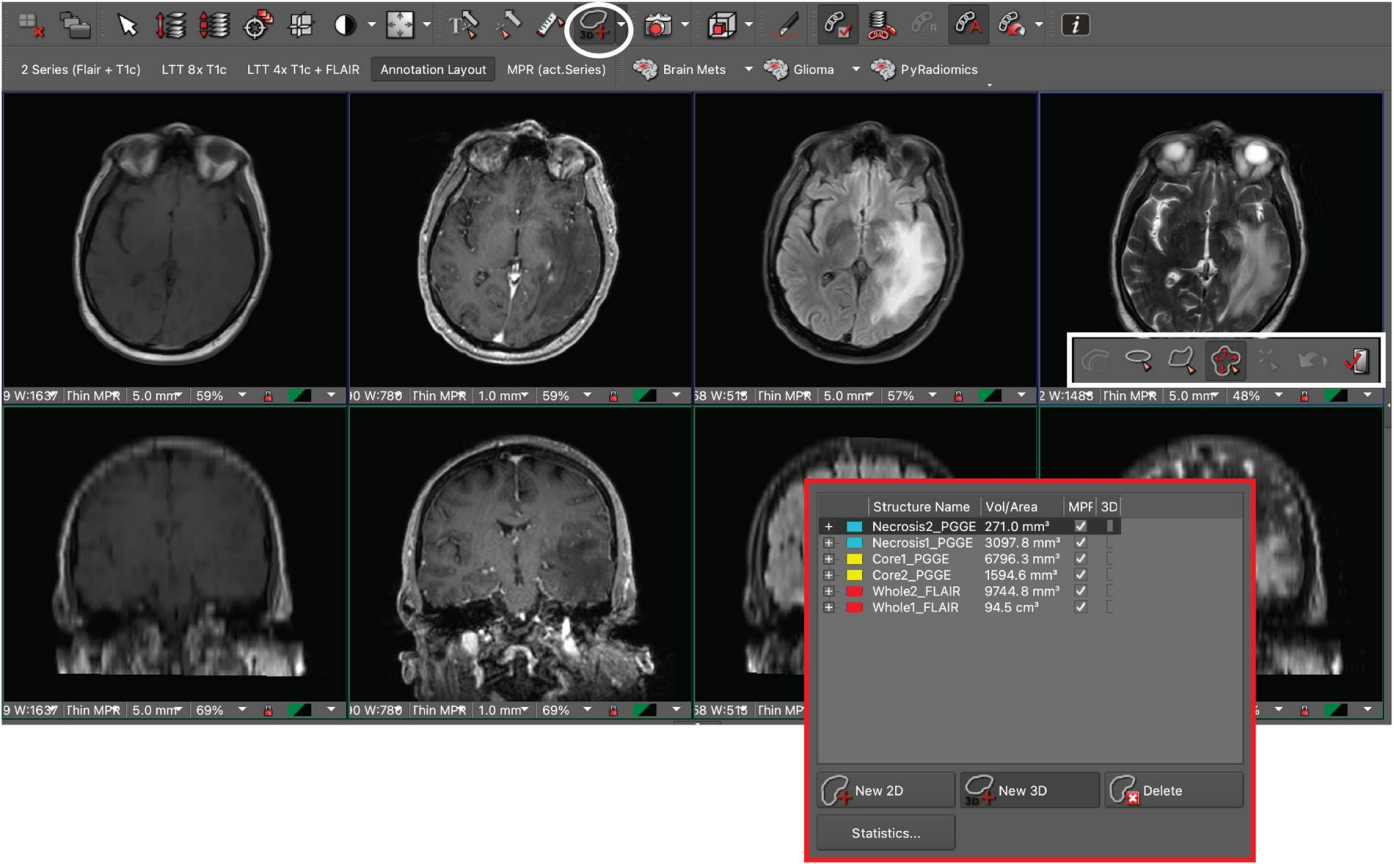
Research PACS annotation layout. The T1, T1 post-contrast, FLAIR, and T2 sequences for one patient are displayed on the eight-viewer layout after alignment with the auto-align tool. The PACS interface incorporates a 3D volumetric tool (white circle/rectangle) and displays labeled segmentations for two brain metastases in the display window (red rectangle).
